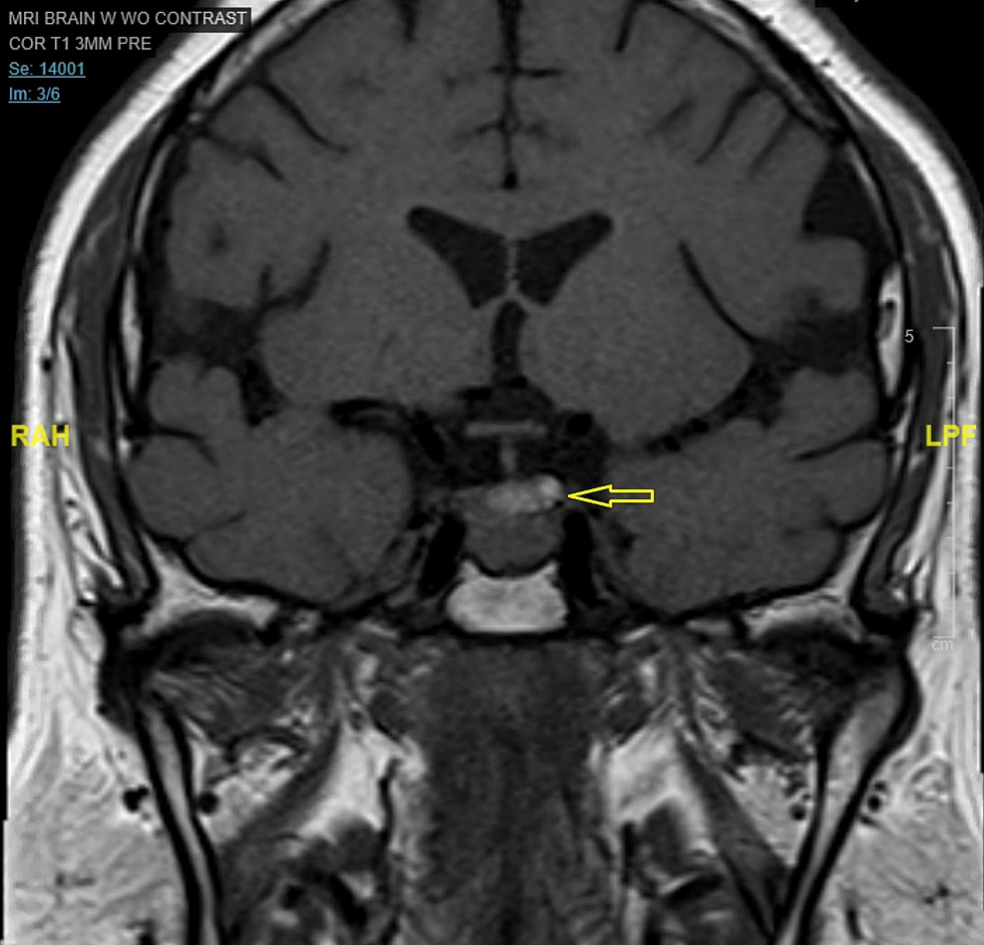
MRI of the sella-turcica revealed a hypodense lesion (yellow arrow) in the inferior pole of the Sella without a supra-sellar extension
